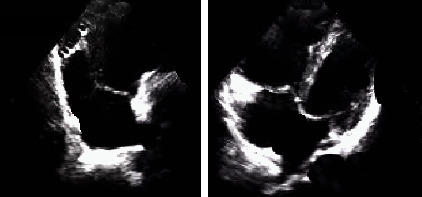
Echocardiograms of a patient with heart failure. Male, 65 years old.
